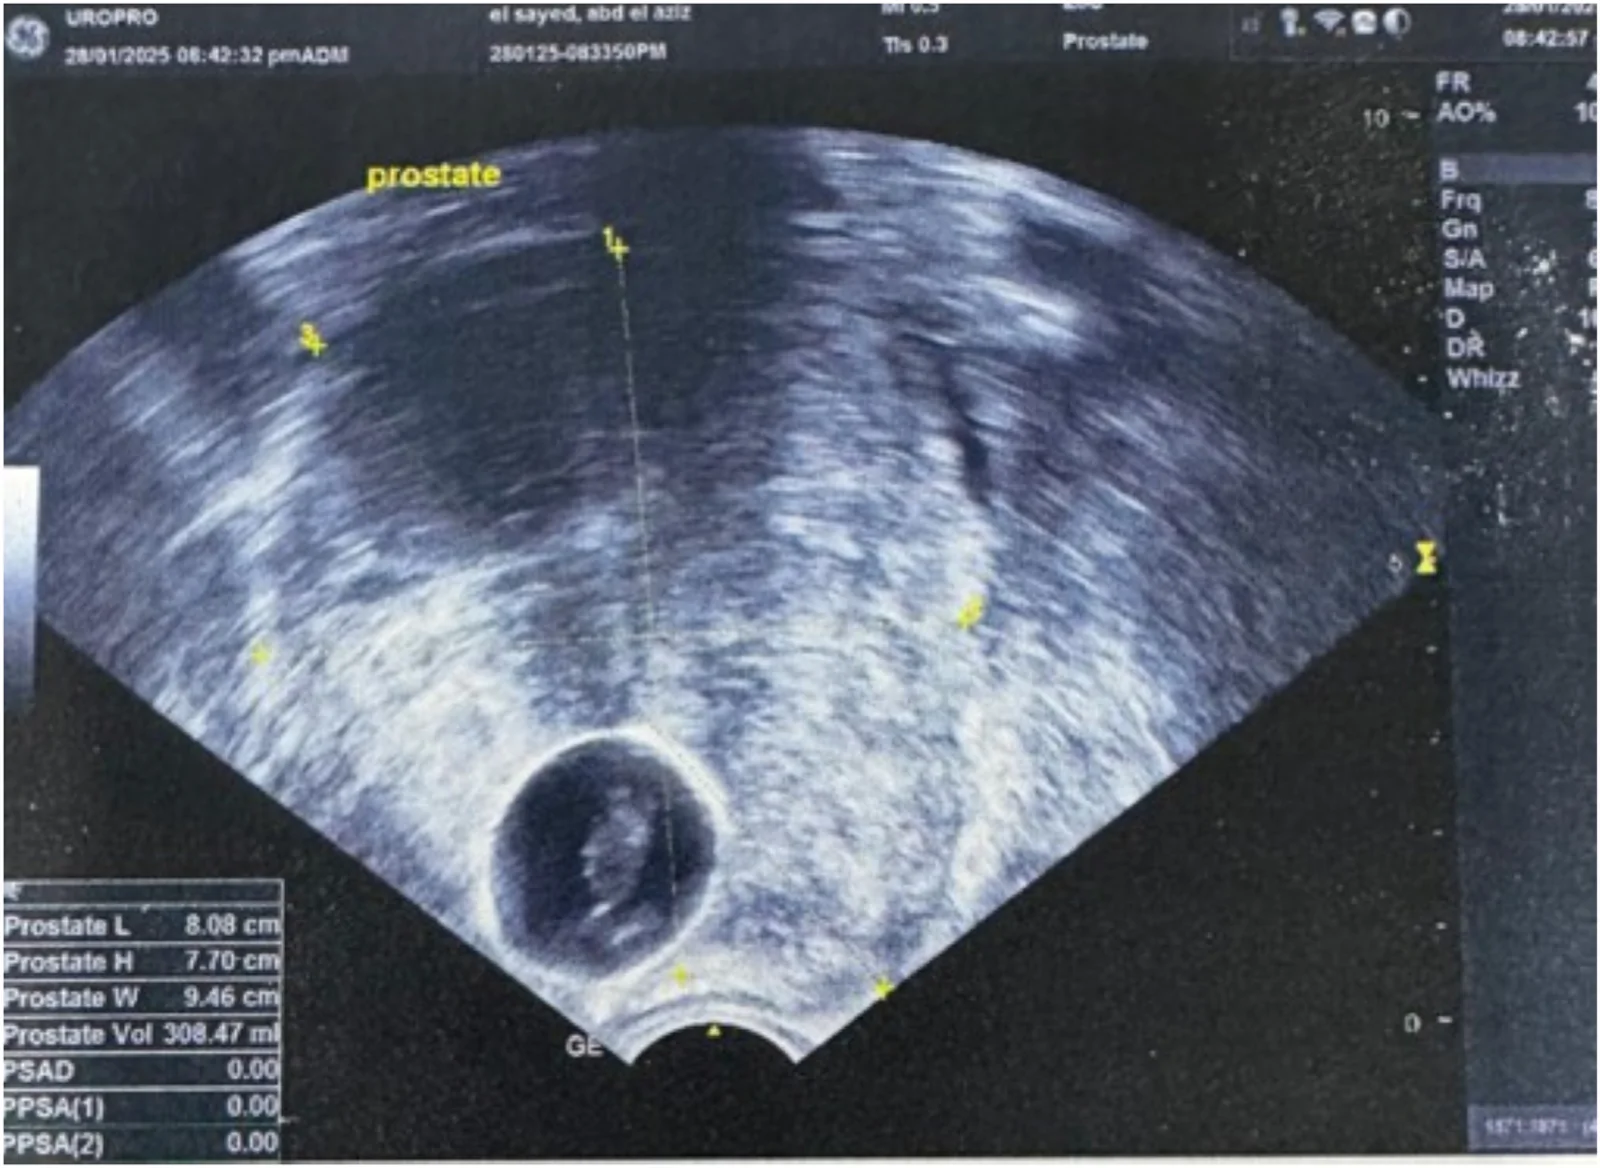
Sonography of the patient demonstrating Giant Benign Prostatic Hyperplasia (BPH) with a calculated volume of 308 mL.
